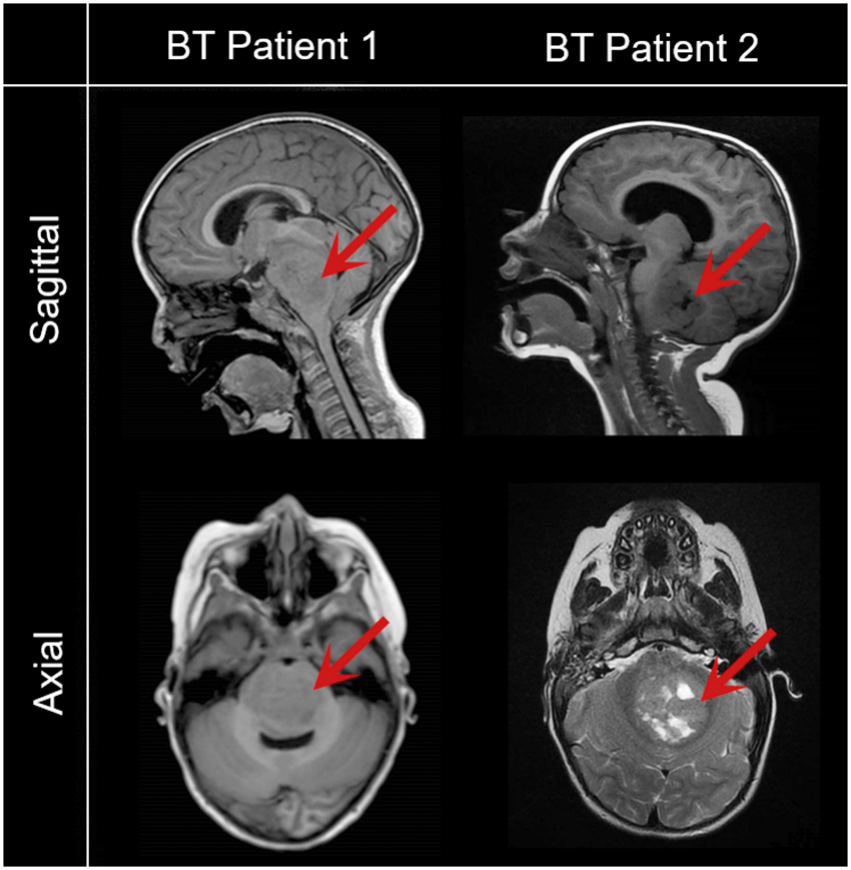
Representative magnetic resonance images of pediatric patients with brain tumors included in the study. Saggital and axial images reveal a low grade glioma (BT Patient 1, 43-month-old) and a metastatic brain tumor (BT Patient 2, 24-month-old). Red arrows point to the tumor.
